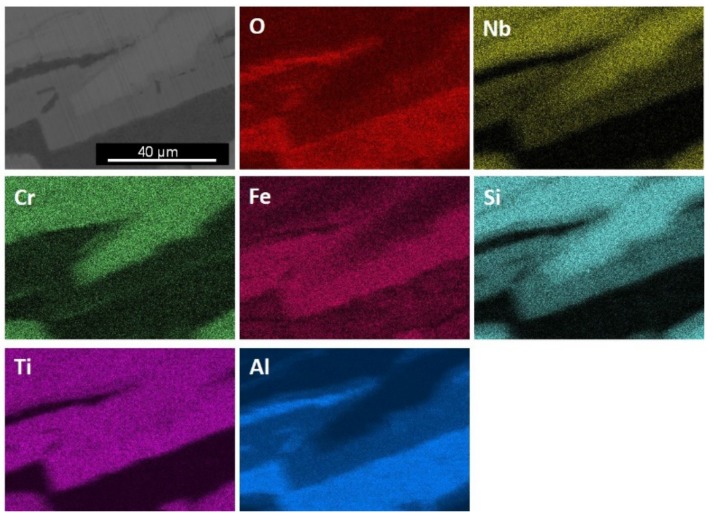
X-ray elemental maps of scale surface after isothermal oxidation at 800 °C for 100 h, BSE image.
